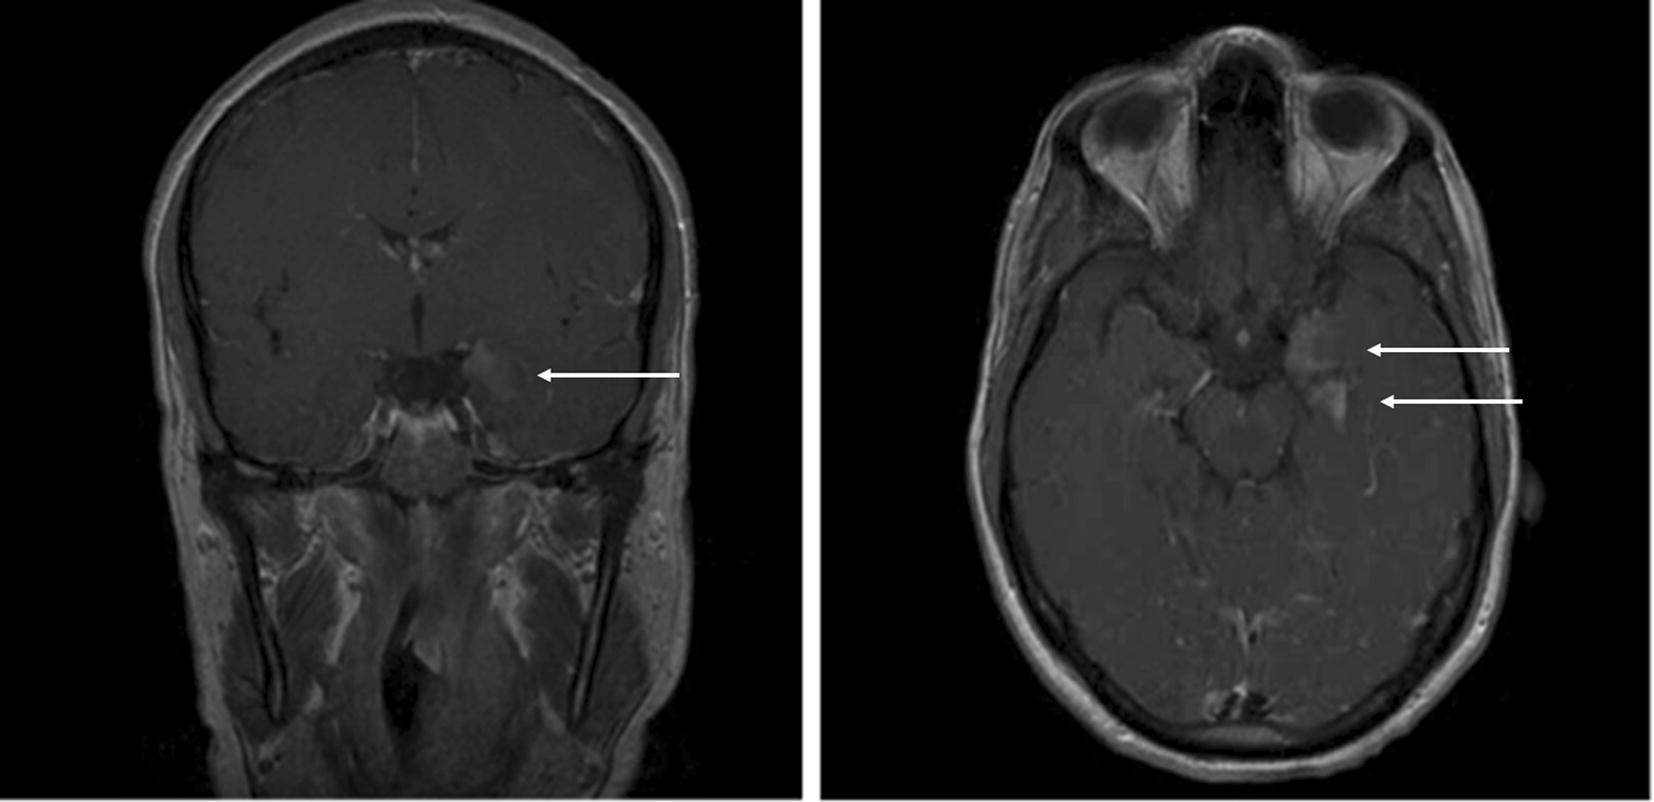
T1 gadolinium-enhanced MRI sequences at day 48 (case 2). Left limbic and hippocampal hypersignals are indicated with white arrows
